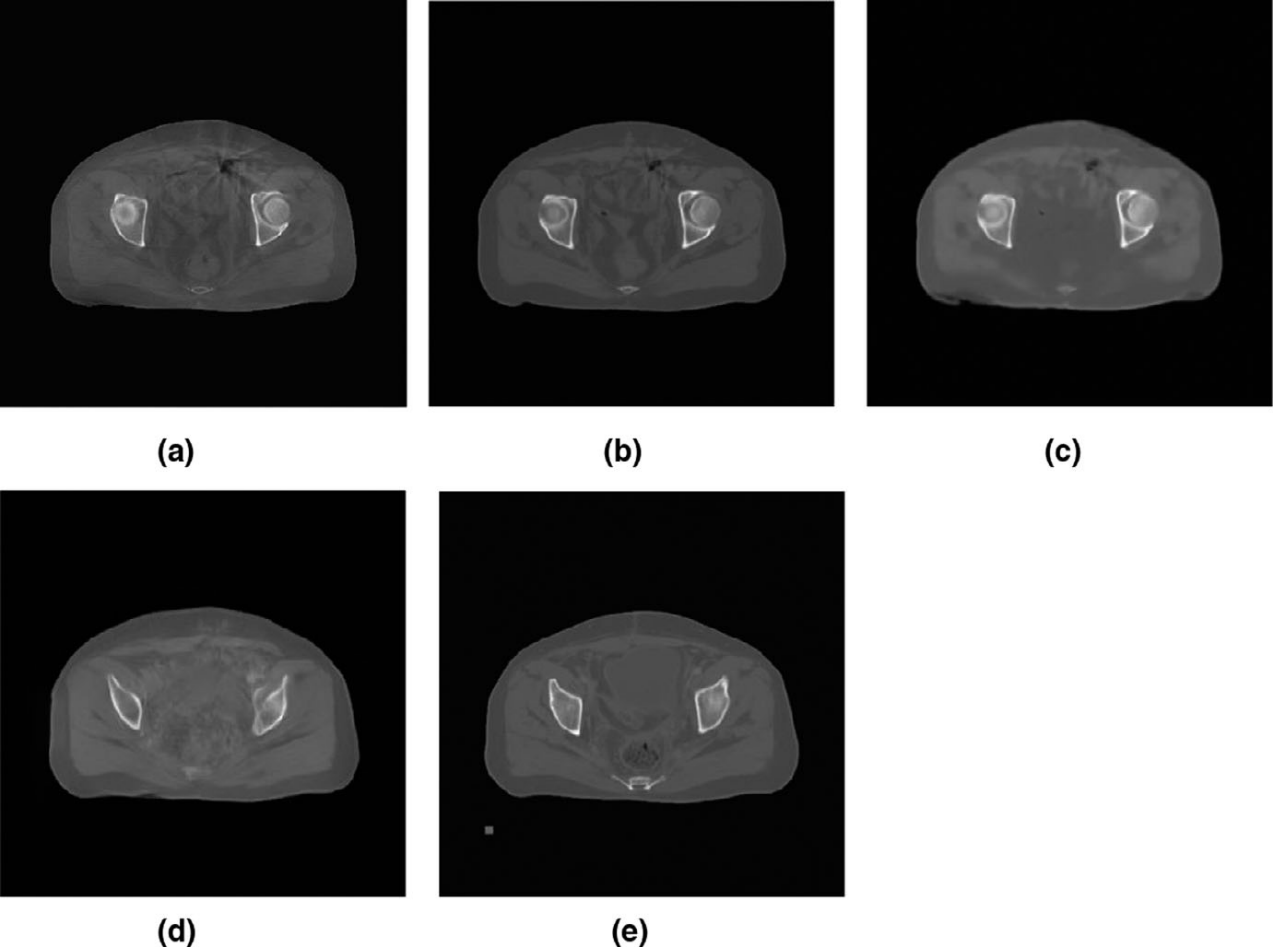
Artifact removal results with different loss function. (a) CBCT, (b) Contextual loss, (c) L2 loss, (d)Perceptual loss, and (e) RCT. Display window [−502, 528].
